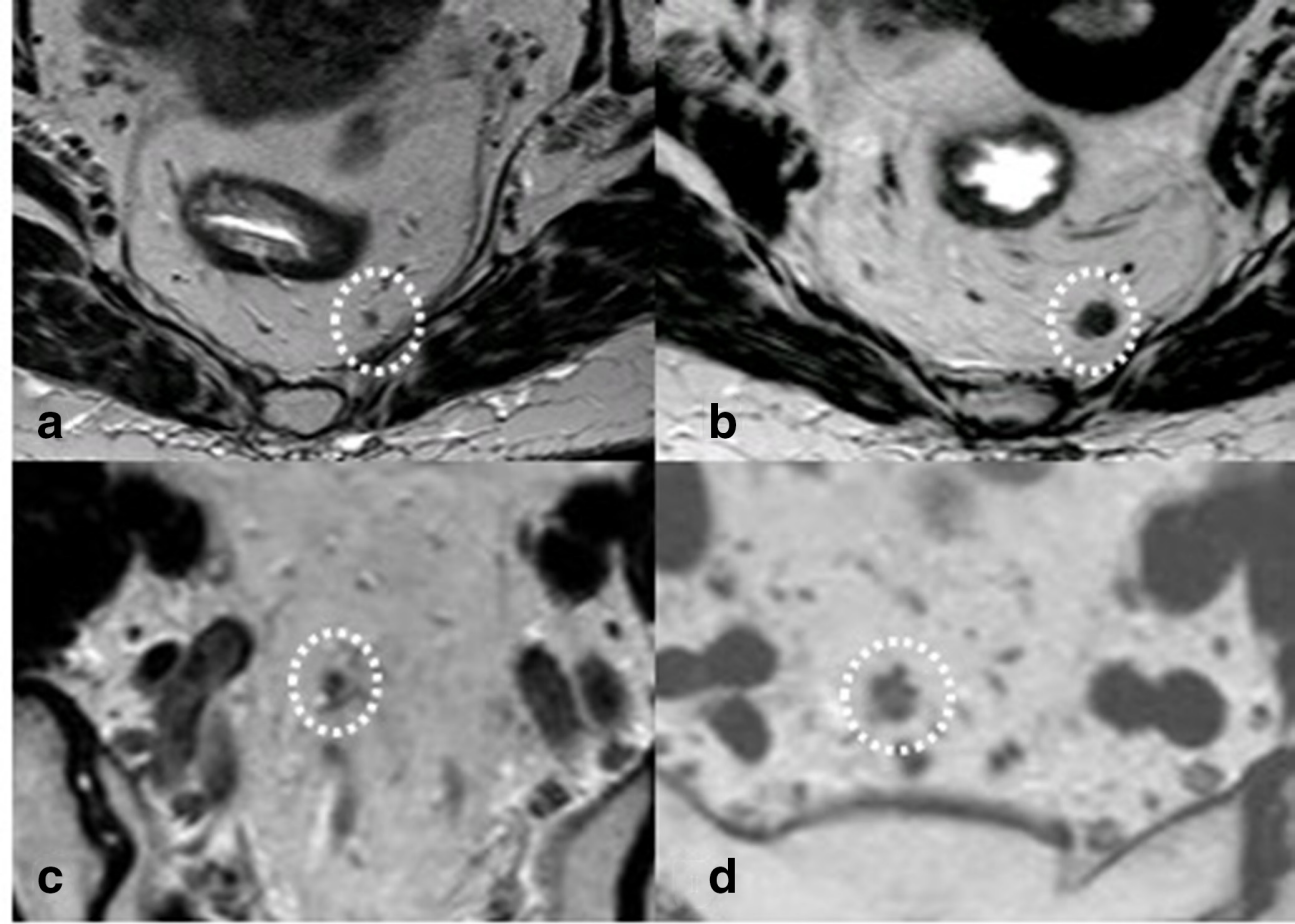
Two patients with nodal regrowths. Patient 1: in (a), the restaging post-CRT T2-weighted image is shown of a node that was malignant but became normalized after treatment. In (b), evident growth is visible. Patient 2: small residual node after CRT in (c) which grows and becomes morphologically suspect during follow-up (d). CRT, chemoradiotherapy.
